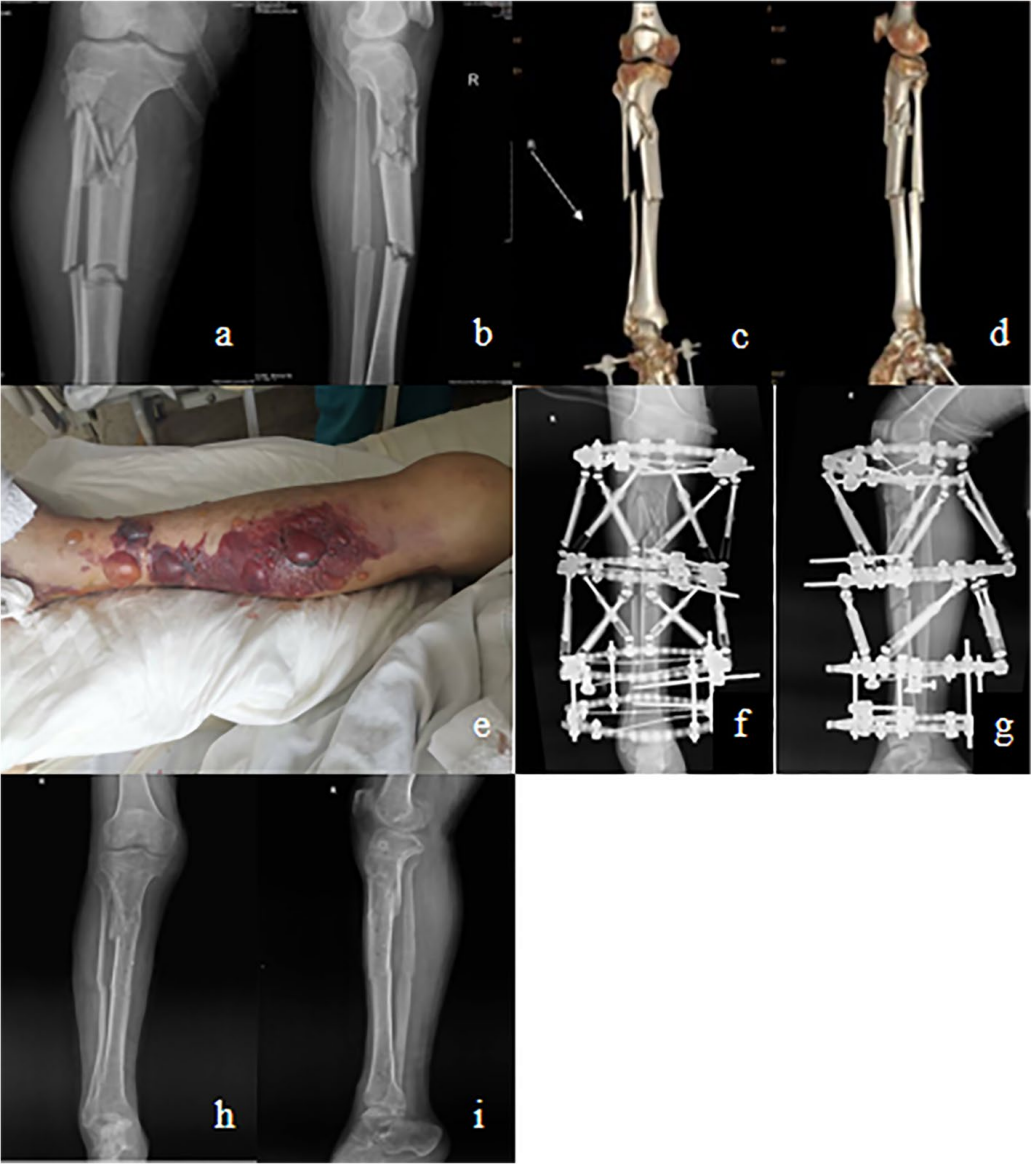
Images of treating a 45-year-old male with STFs due to traffic accident by using HEF. a, b X-ray images of injured STFs preoperatively. c, d Computer tomography images of injured STFs preoperatively. e Appearance image shows poor local soft tissue conditions in the right lower leg preoperatively. f, g X-ray images within one week postoperatively. h, i X-ray images show good fracture healing, one week after removal of HEF
